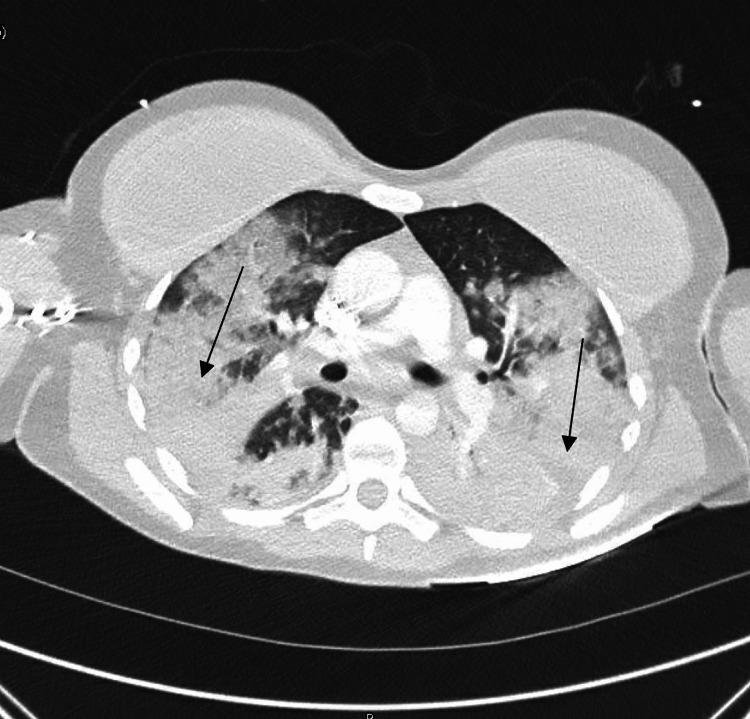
CTA of the chest reveals extensive bilateral consolidation, which may represent pneumonia, pulmonary hemorrhage, or acute respiratory distress syndrome. No evidence of pulmonary embolism. CTA, computed tomography angiography
